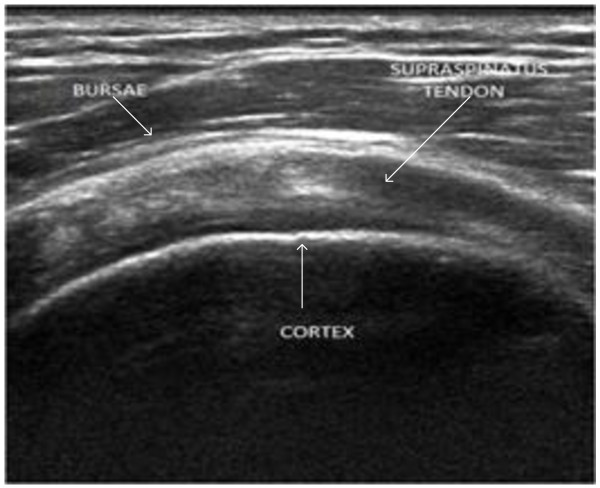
SSP tendon ultrasound image.
